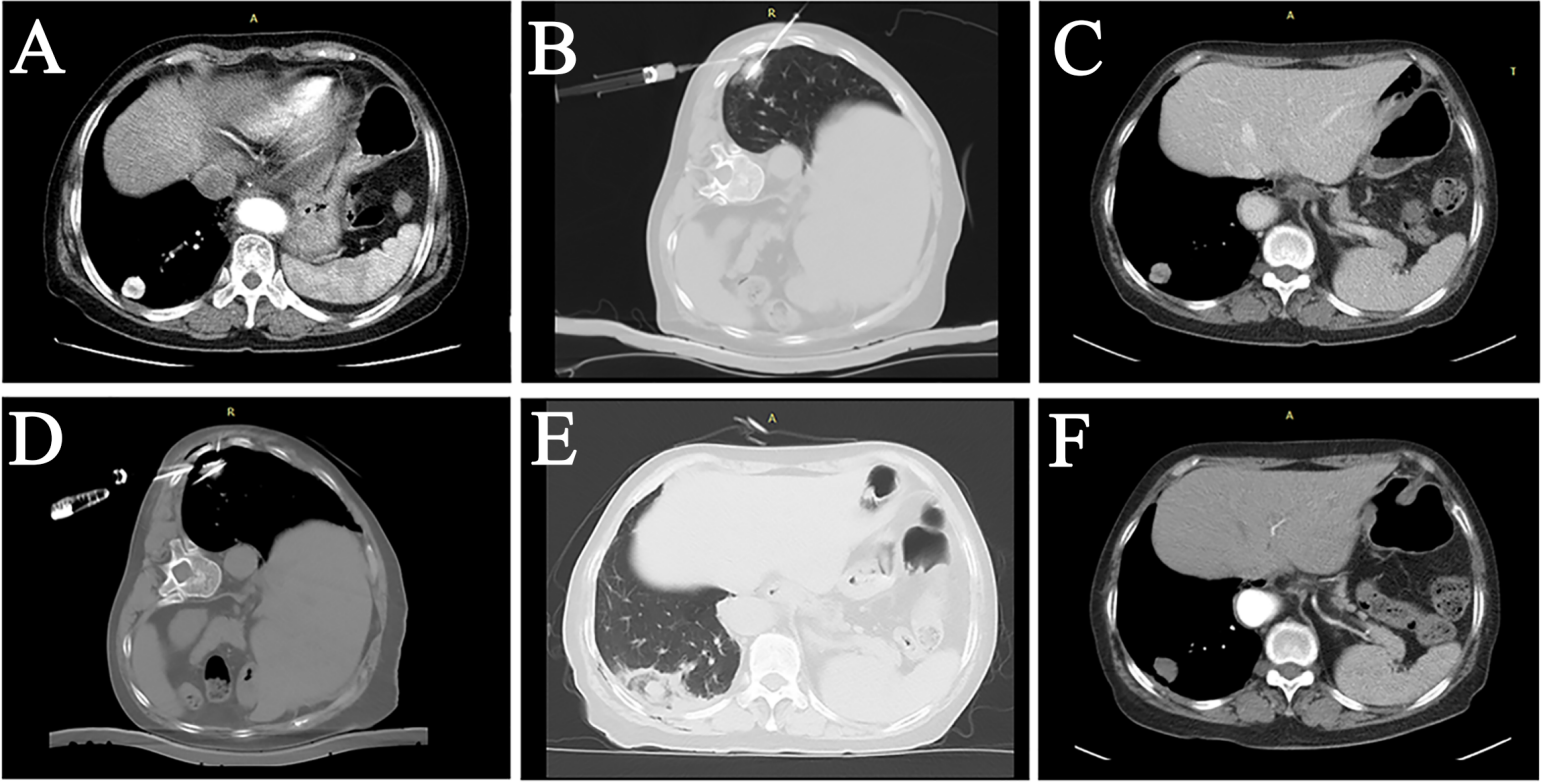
A female patient, 72 years old, with lung adenocarcinoma in the right lower lung lobe, underwent secondary ablation after the first incomplete ablation. (A) The tumor was located in the subpleural area with significant enhancement. (B) First MWA treatment combined with LPA. (C) Follow-up CT three months after the intervention showed significant inhomogeneous enhancement within the tumor. (D) Re-ablation of the tumor was performed using two MWA antennas combined with LPA. (E) Postoperative CT showed a “halo sign” surrounding the tumor. (F) 3-month follow-up after the secondary ablation indicated complete tumor ablation.
